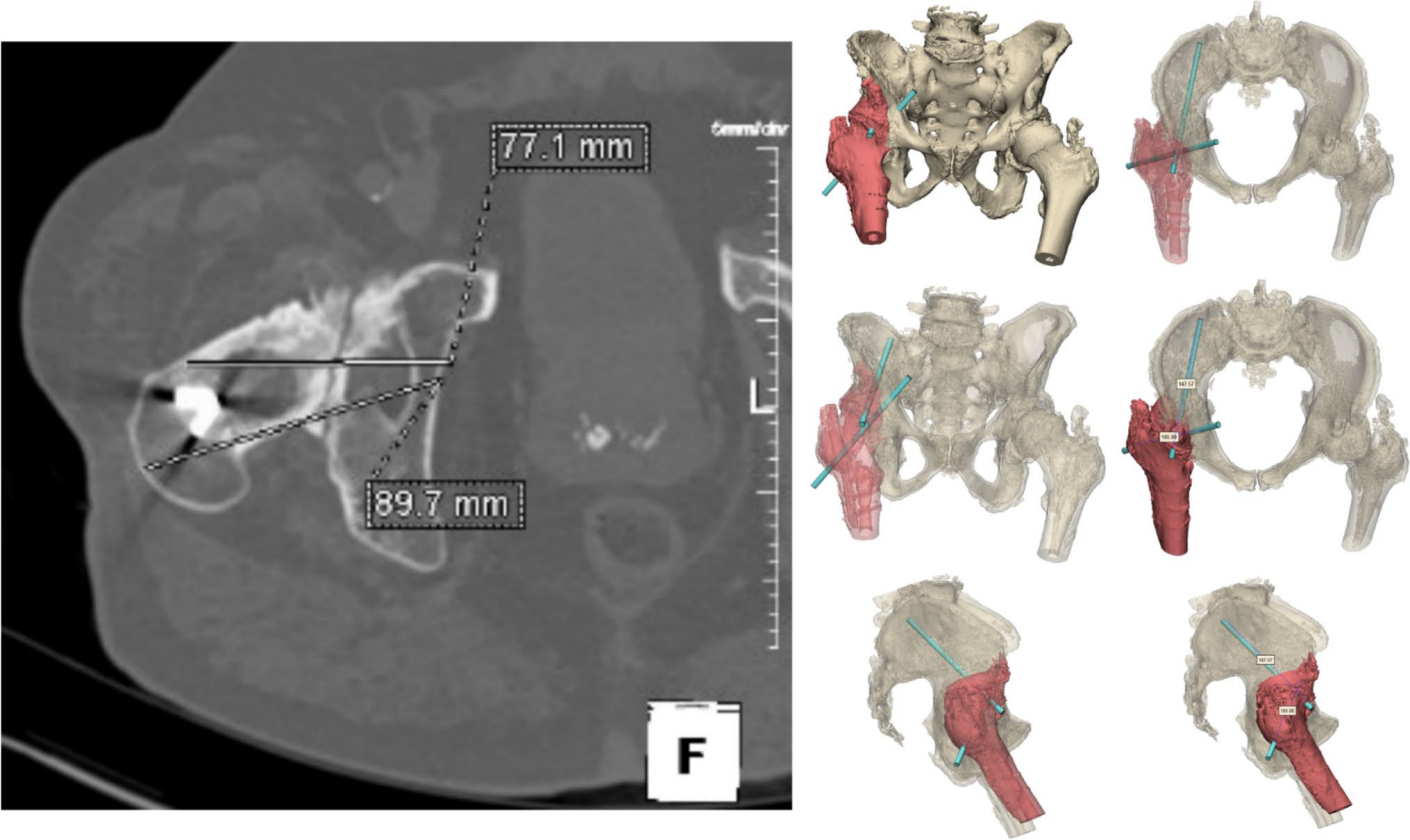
Case 2. Preoperative CT and 3D planning of percutaneous minimal invasive screws around the already existing plate
